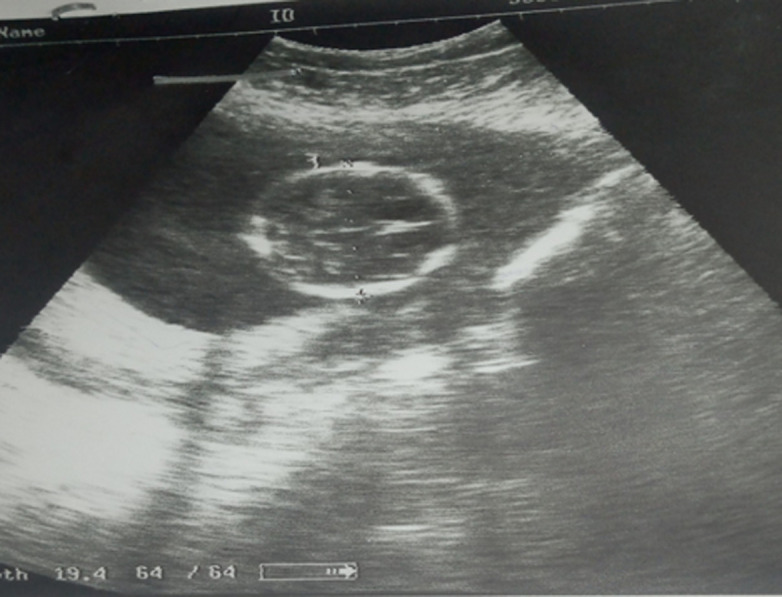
second ultrasound performed: gestational sac with thick wall without fetal anomaly or appendages; next to a non-gravid but globular uterus
